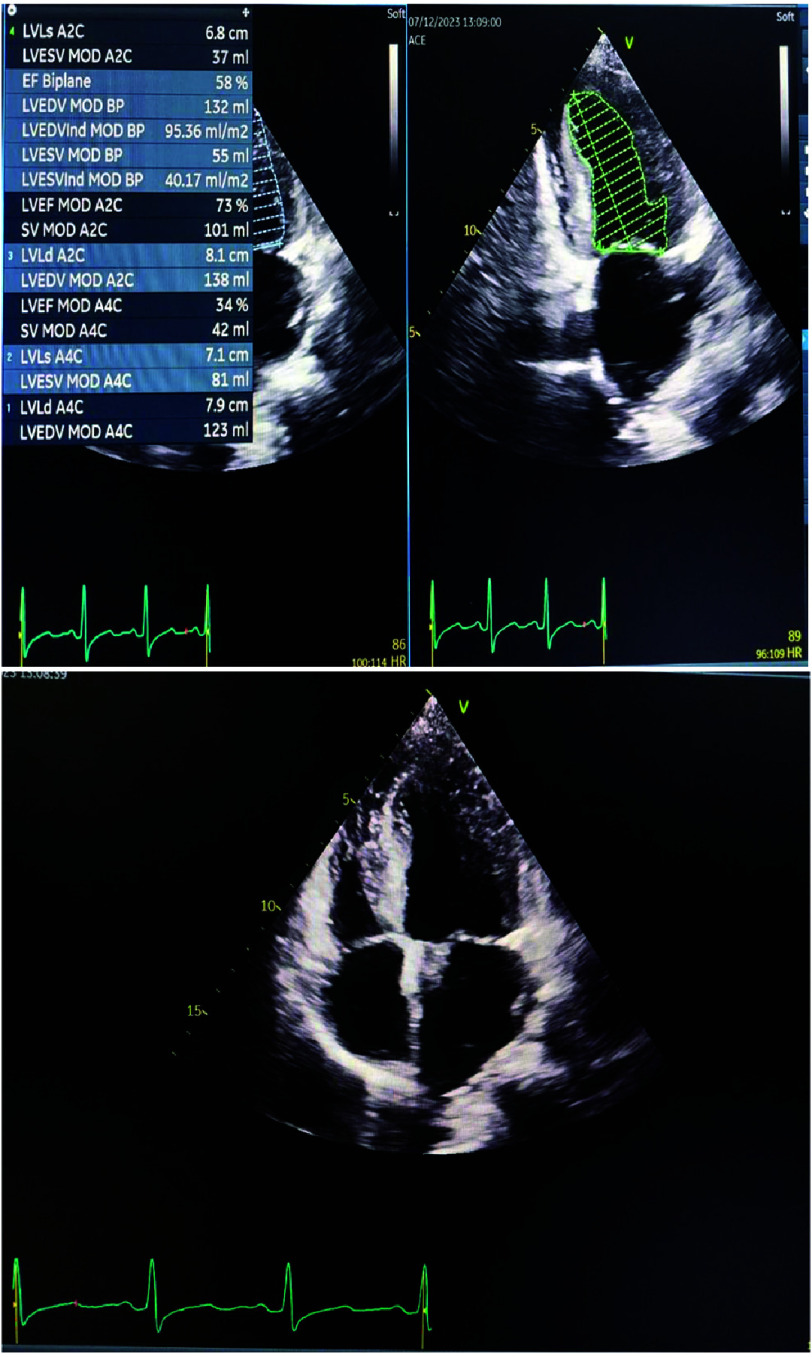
Apical two-chamber and four-chamber echocardiography. The ejection fraction (EF) was calculated in biplane mode (result 58%). Granular-sparkling appearance was evident in both the apical two-chamber and four-chamber echocardiographic views.
